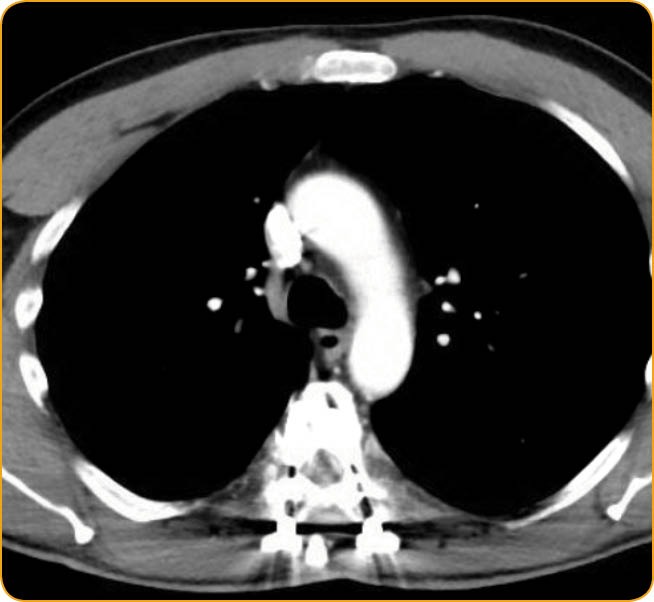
CT scan shows a normal-looking mediastinum.
